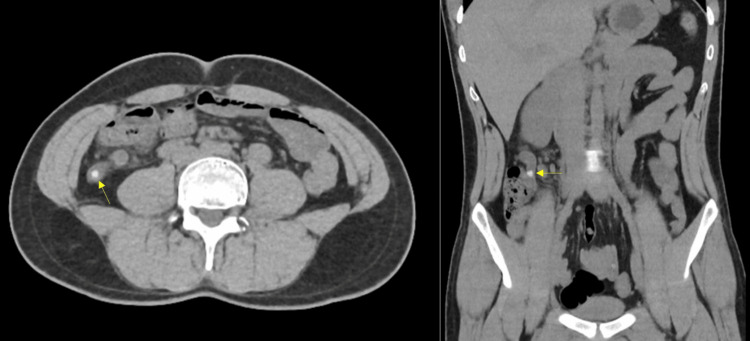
Abdominal CT Showing Left-Sided Retrocecal Appendix With Fecolith (Arrow) and Significantly Increased Diameter. CT, computed tomography
